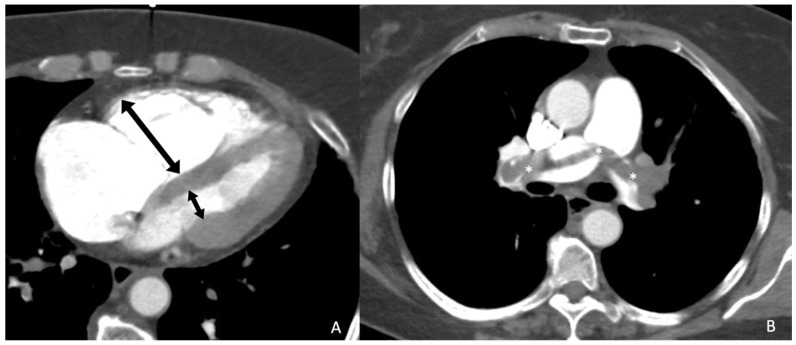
Fifty-six-year-old woman diagnosed with acute pulmonary thromboembolism, by axial CT angiography. (A). Axial RV/LV diameter ratio > 1 measured at the base of both ventricles (black arrows). (B). Filling defects in both main pulmonary arteries (*), with a saddle thrombus.
